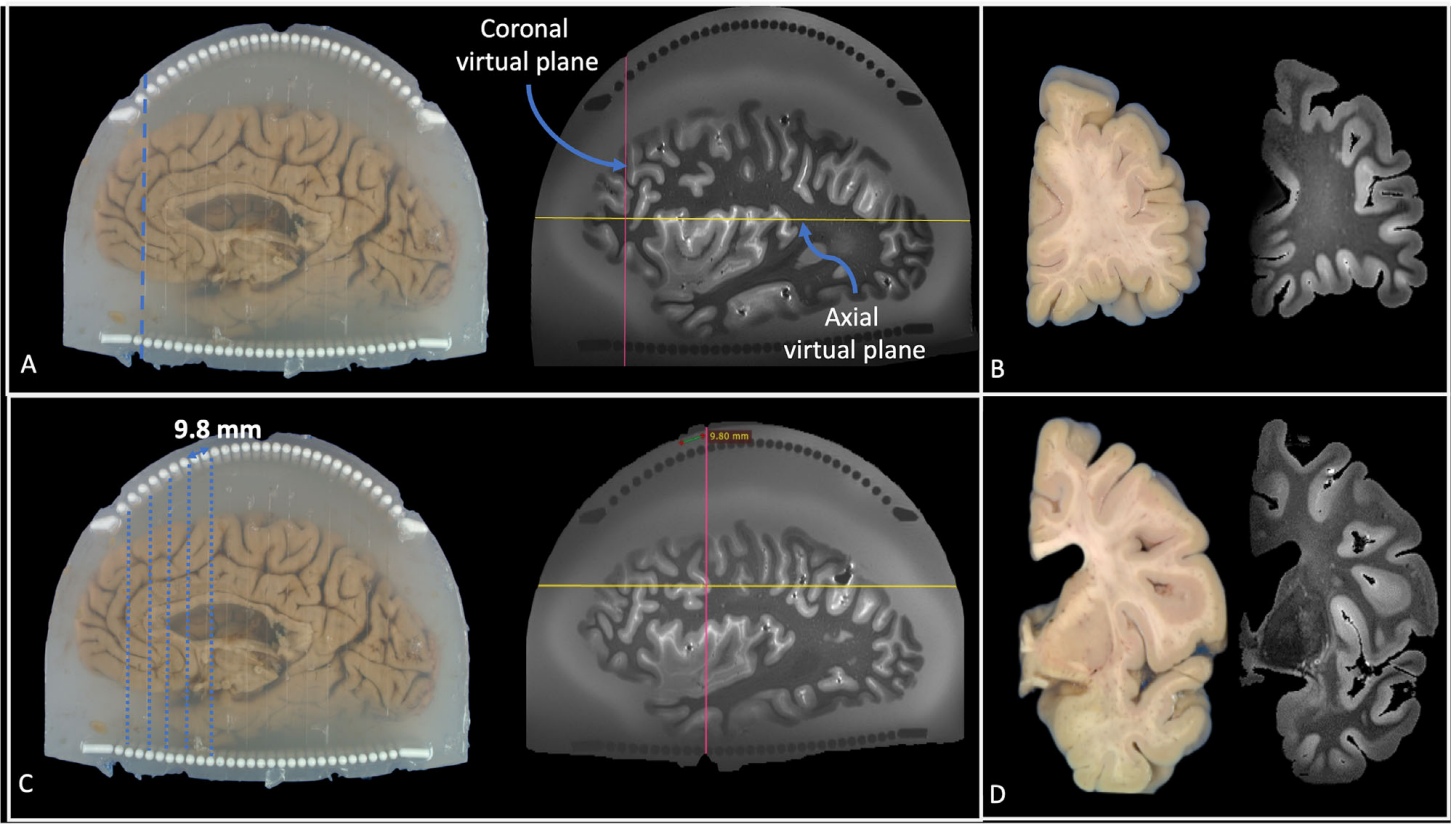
Alignment of MRI virtual cutting planes with knife traces on blockface photographs. (A) After sectioning the brain using the cutting guides, knife traces become visible on the agarose surface and are manually highlighted with a dashed blue line. The pink line represents the coronal virtual plane used for alignment, whereas the yellow line represents the axial virtual plane (shown for reference but not used during the alignment process). During alignment, the coronal virtual plane is oriented to match the knife trace and positioned to pass through the corresponding column pair. (B) During alignment, each MRI coronal slice is visually matched to the corresponding blockface photograph before proceeding to the next slice. (C) As alignment progresses, previously matched knife traces are marked with thin blue dashed lines, whereas the current slice position is indicated by a thicker blue dashed line. The pink coronal plane shows the current MRI reconstruction plane, aligned with the cutting orientation. The yellow line indicating the axial plane is shown for reference but not used during the alignment process. (D) The visual match between MRI and blockface images is confirmed for each slab before advancing to the next cutting plane.
